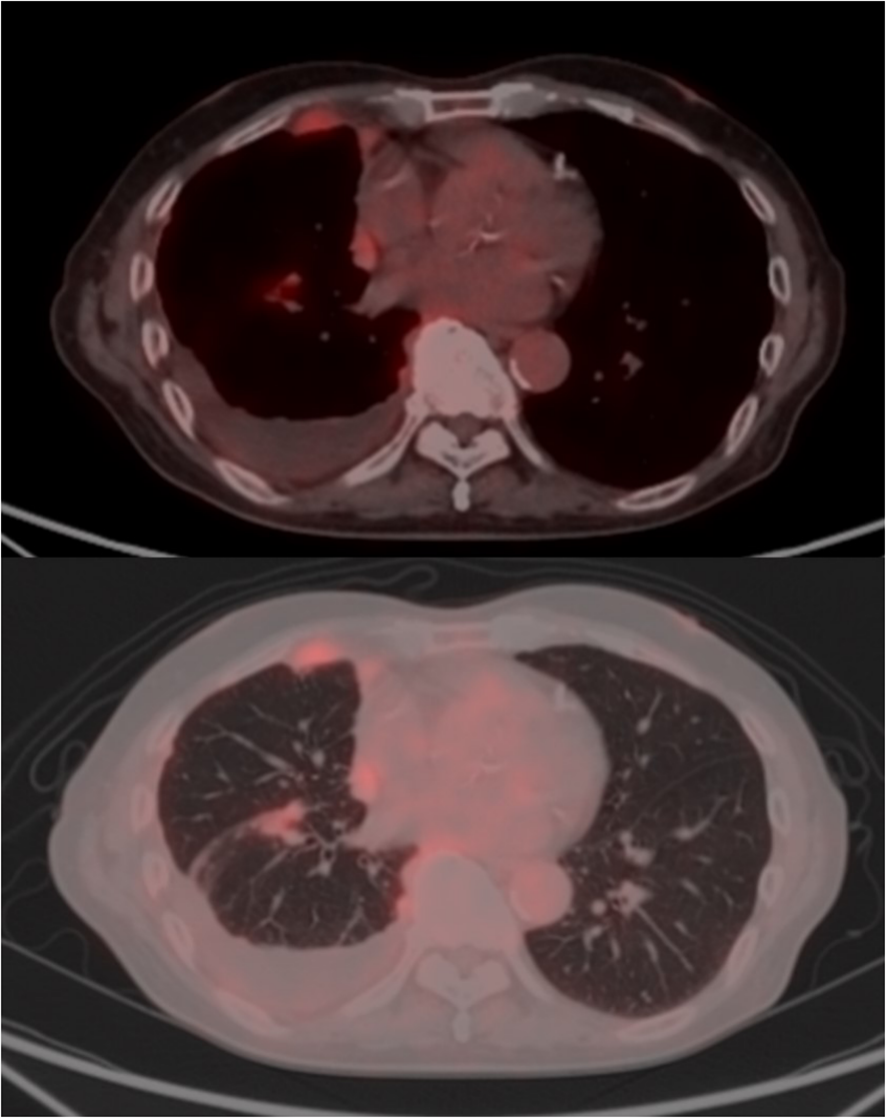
Radiological findings of malignant pleural mesothelioma. Positron emission tomography (PET) revealed asymmetric thickening of the right pleura, including the interlobar pleura, with heterogeneous fluorodeoxyglucose (FDG) uptake (peak SUVmax 4.0). Abnormal FDG accumulation was also observed in an enlarged lymph node-like structure located anterior to the right brachiocephalic vein and contiguous with the thickened pleura. Additionally.
